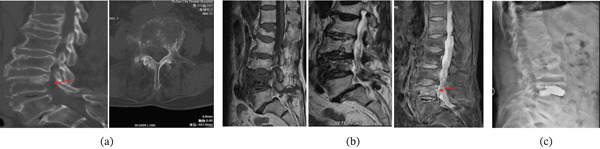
Imaging and clinical course of the patient in Case 3 (brucellar spondylitis misdiagnosed as Kümmell disease). (a) Preoperative sagittal CT showed multilevel vertebral height loss. Axial CT at L4 revealed subtle marginal bone erosion without obvious sequestrum formation—a pattern initially attributed to the nonunion of Kümmell disease rather than infection. (b) MRI showed an intravertebral cleft‐like signal at L4, which further reinforced the erroneous diagnosis of avascular necrosis. (c) Postoperative lateral radiograph after L4 PVP showed satisfactory cement filling. Brucellar spondylitis was later confirmed by positive blood culture for *Brucella melitensis* and a positive Rose Bengal plate test.
